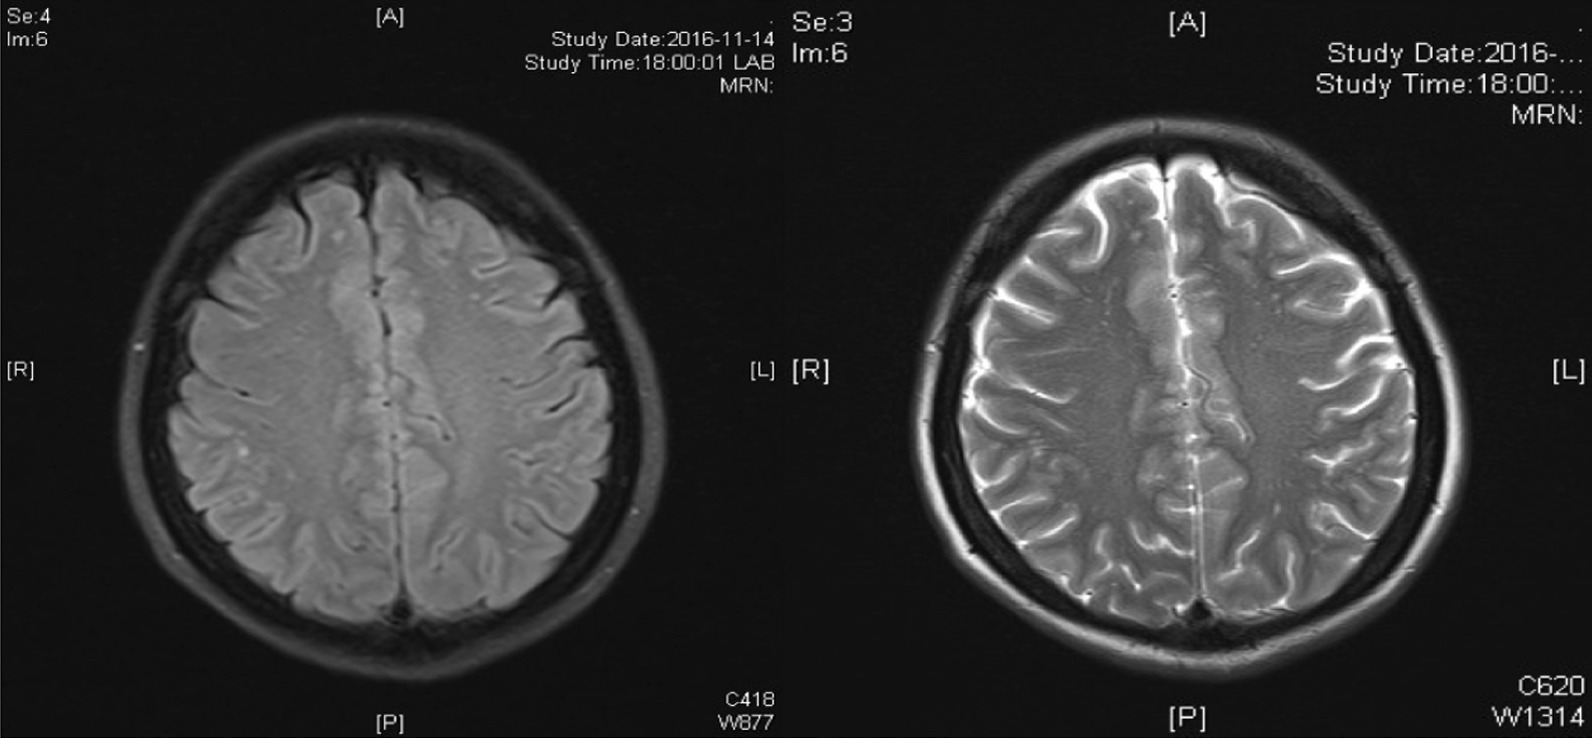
Cranial MRI revealing multiple demyelinating lesions in the white matter of both cerebral hemispheres
